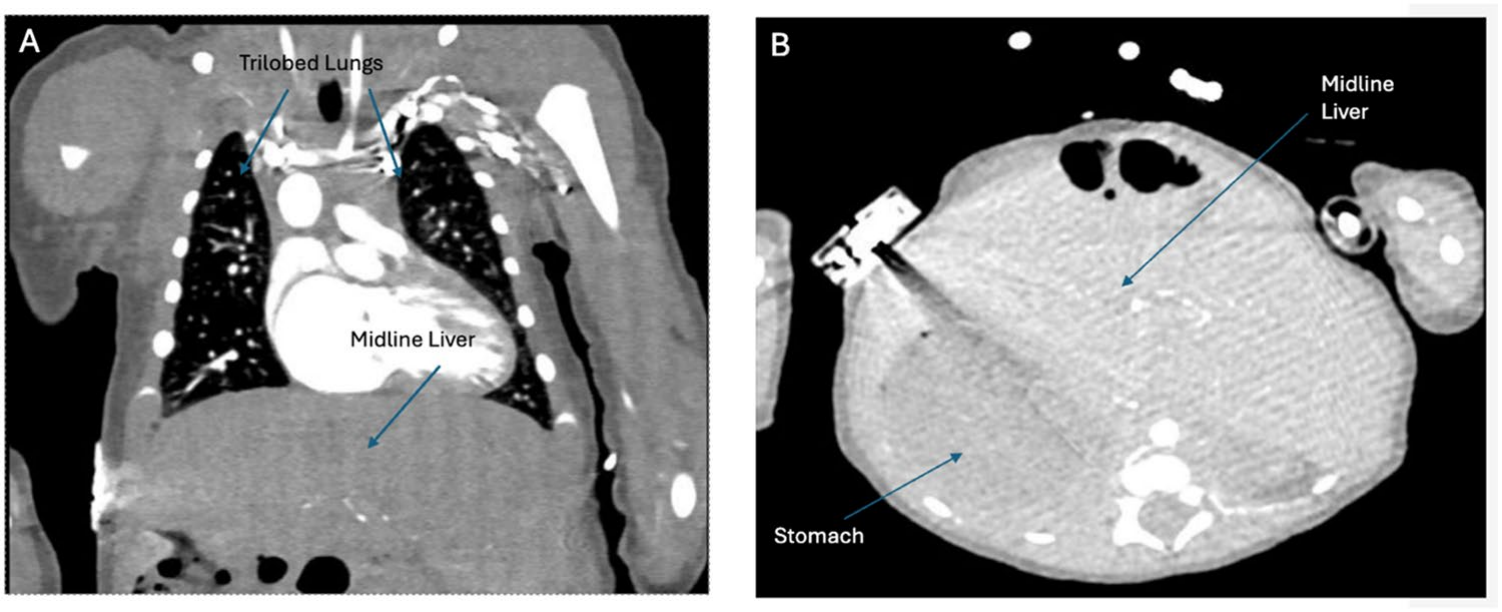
(A) Computed tomography angiography of the chest showing midline liver and tri-lobed right lungs bilaterally. (B) Transverse computed tomography scan showing midline liver and abnormal stomach placement
